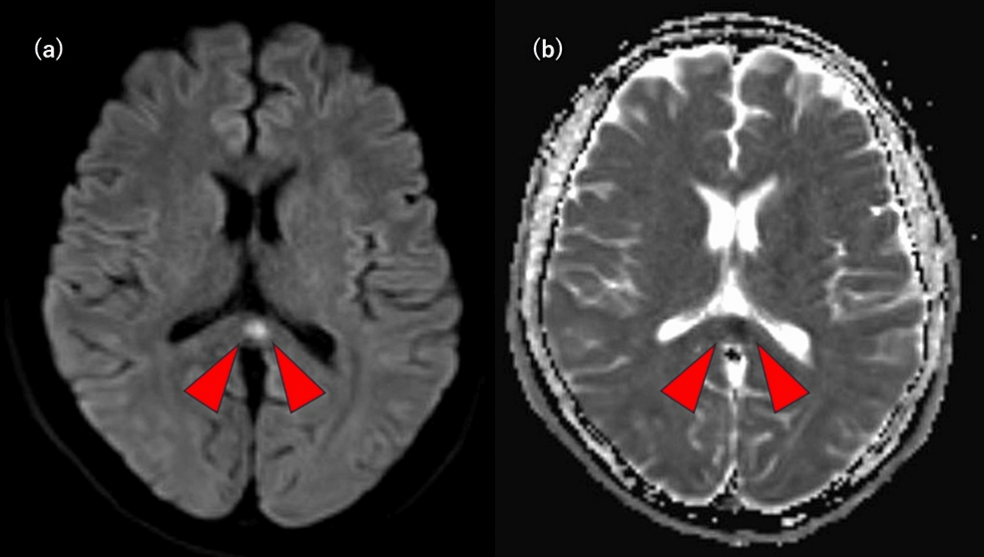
A head MRI image of postoperative day one. (a) DWI image shows symmetrical lesions with high signal in the corpus callosum (red arrowhead). (b) ADC map image shows symmetrical lesions with reduced ADC values in the corpus callosum (red arrowhead).
